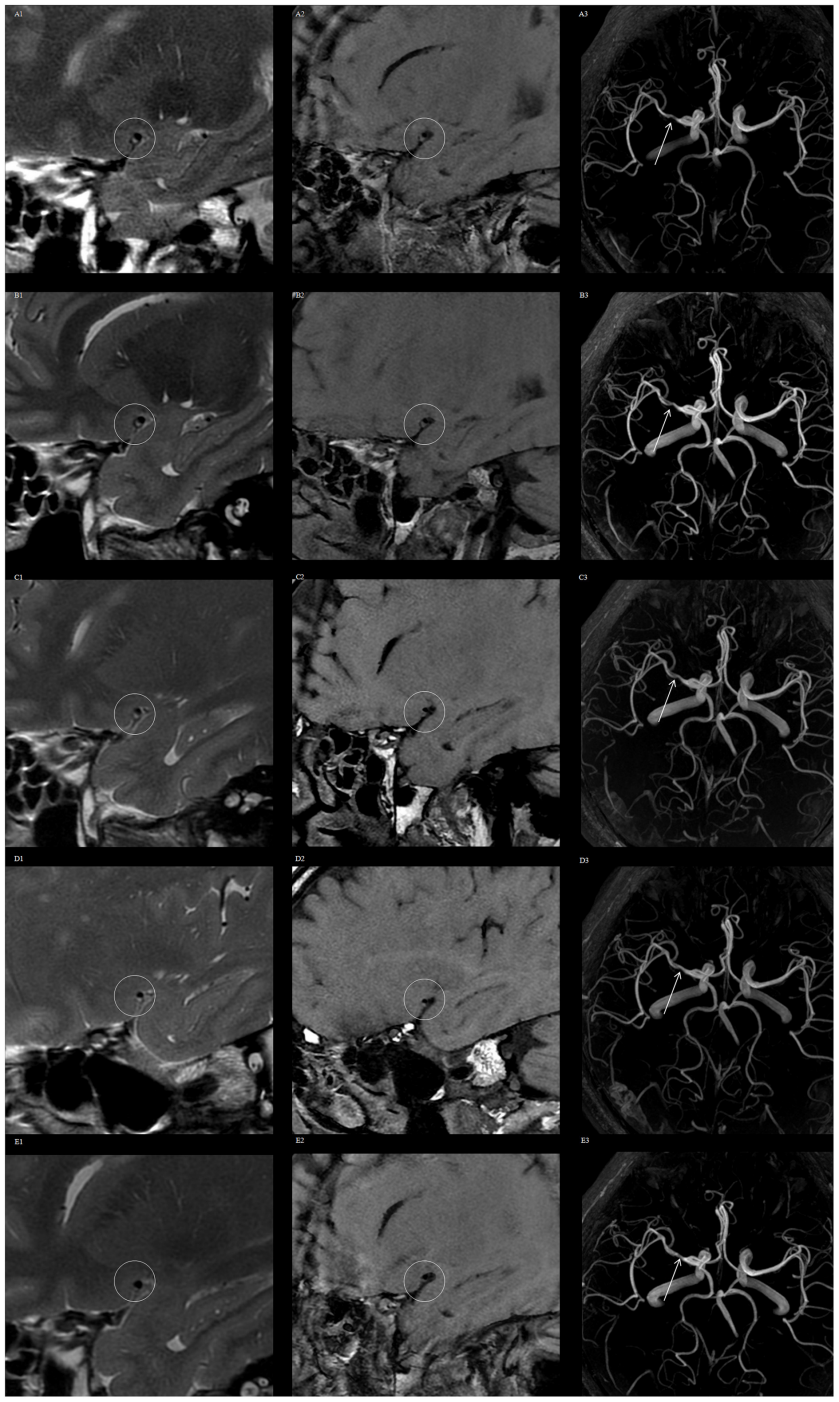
Vessel wall magnetic resonance imaging (VWMRI) of plaque changes in the right middle cerebral artery (MCA) of a patient during the follow-up period. VWMRI and MRA show no significant changes in plaque volume and luminal stenosis at baseline, 1, 3, and 6 months. The plaque volume and luminal stenosis degree were reduced at the 12-month follow-up time point. Baseline, 1-, 3-, 6-, and 12-month 3D CUBE T2 images (A1–E1), 3D CUBE T1 sequence images (A2–E2), and 3D TOF MRA images (A3–E3).
